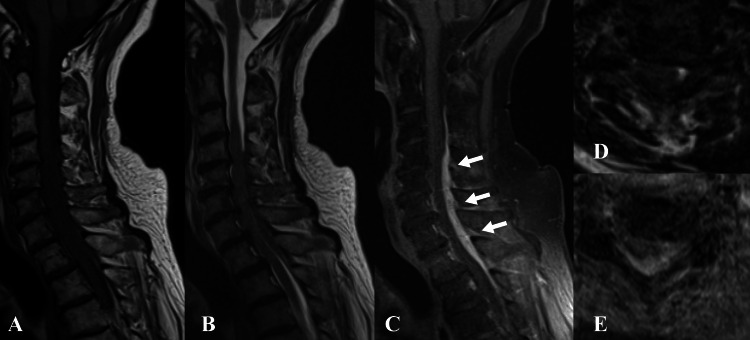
Preoperative gadolinium-enhanced MRI of the cervical spine The spinal cord is severely compressed by a mass lesion dorsally at the level of C4-Th1 (white arrows). The patient was unable to maintain the posture due to the neck pain, which resulted in blurry images. Aː Sagittal T1-weighted image; Bː Sagittal T2-weighted image; Cː Sagittal T1 fat-saturated weighted image; D: Axial plain T2-weighted image at the C4/5 level; E: Axial gadolinium-enhanced image at the C4/5 level
